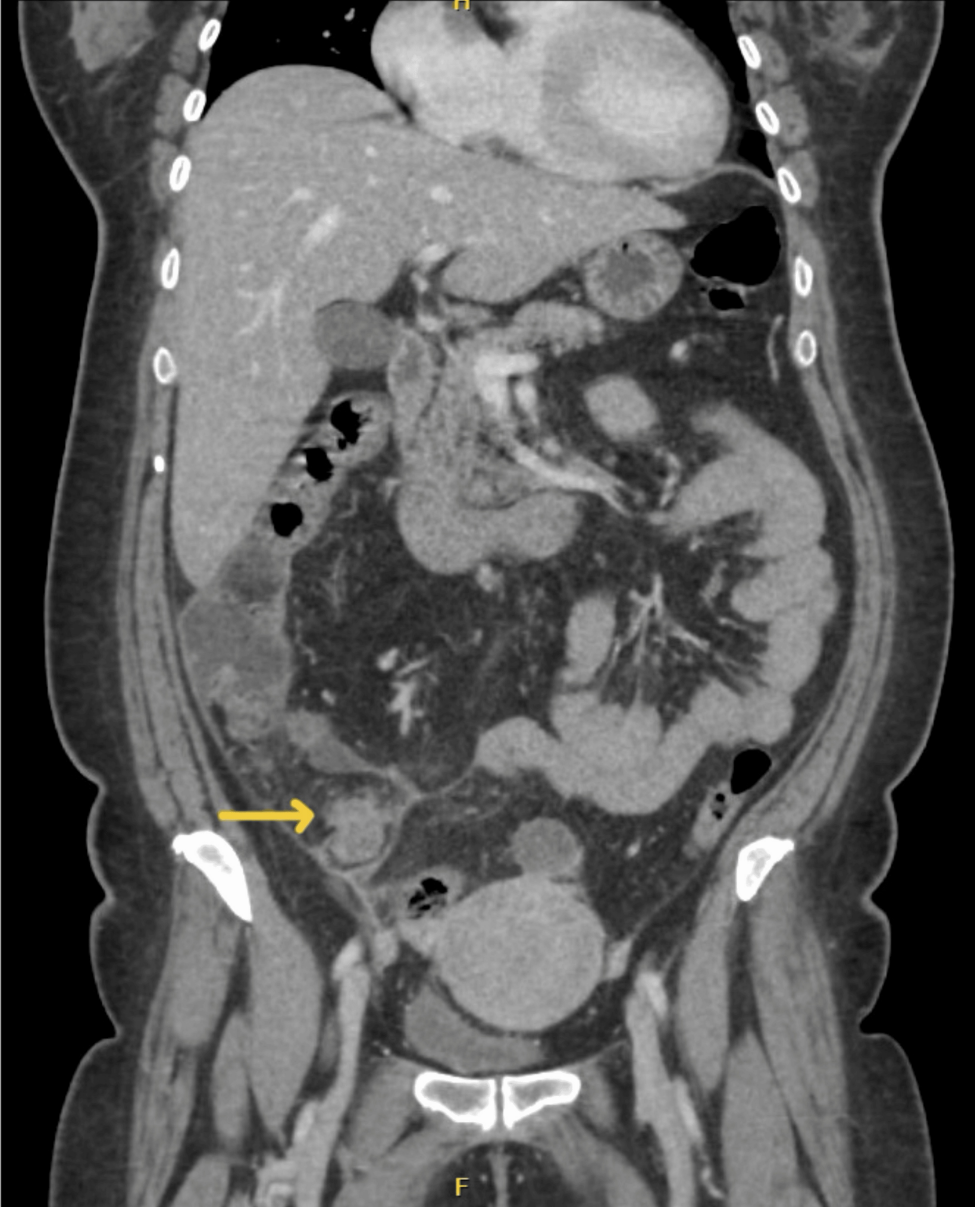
Coronal CT scan of the abdomen and pelvis with IV contrast showing dilated tip of the appendix, around 1.4 cm in diameter, with marked periappendiceal fat stranding and minimal free fluid CT: computed tomography; IV: intravenous
